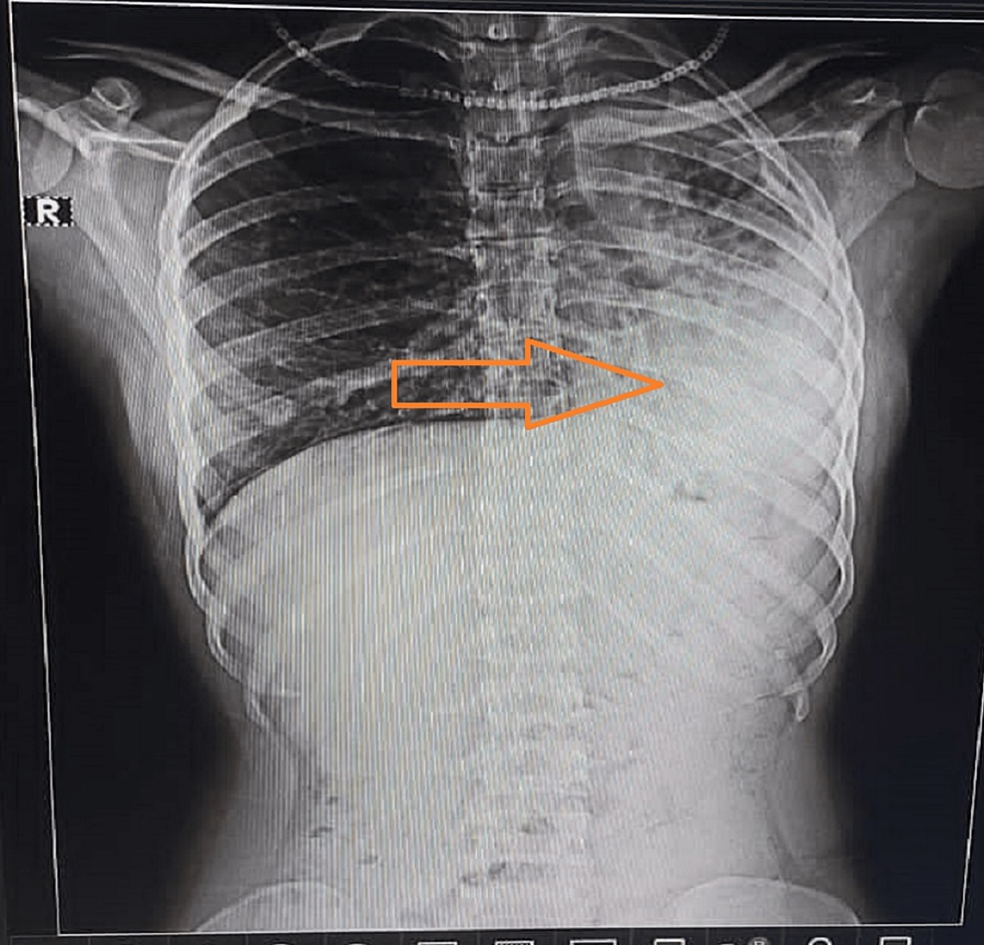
PA view chest X-ray showing left-sided basal lung fibrosis PA: posteroanterior
